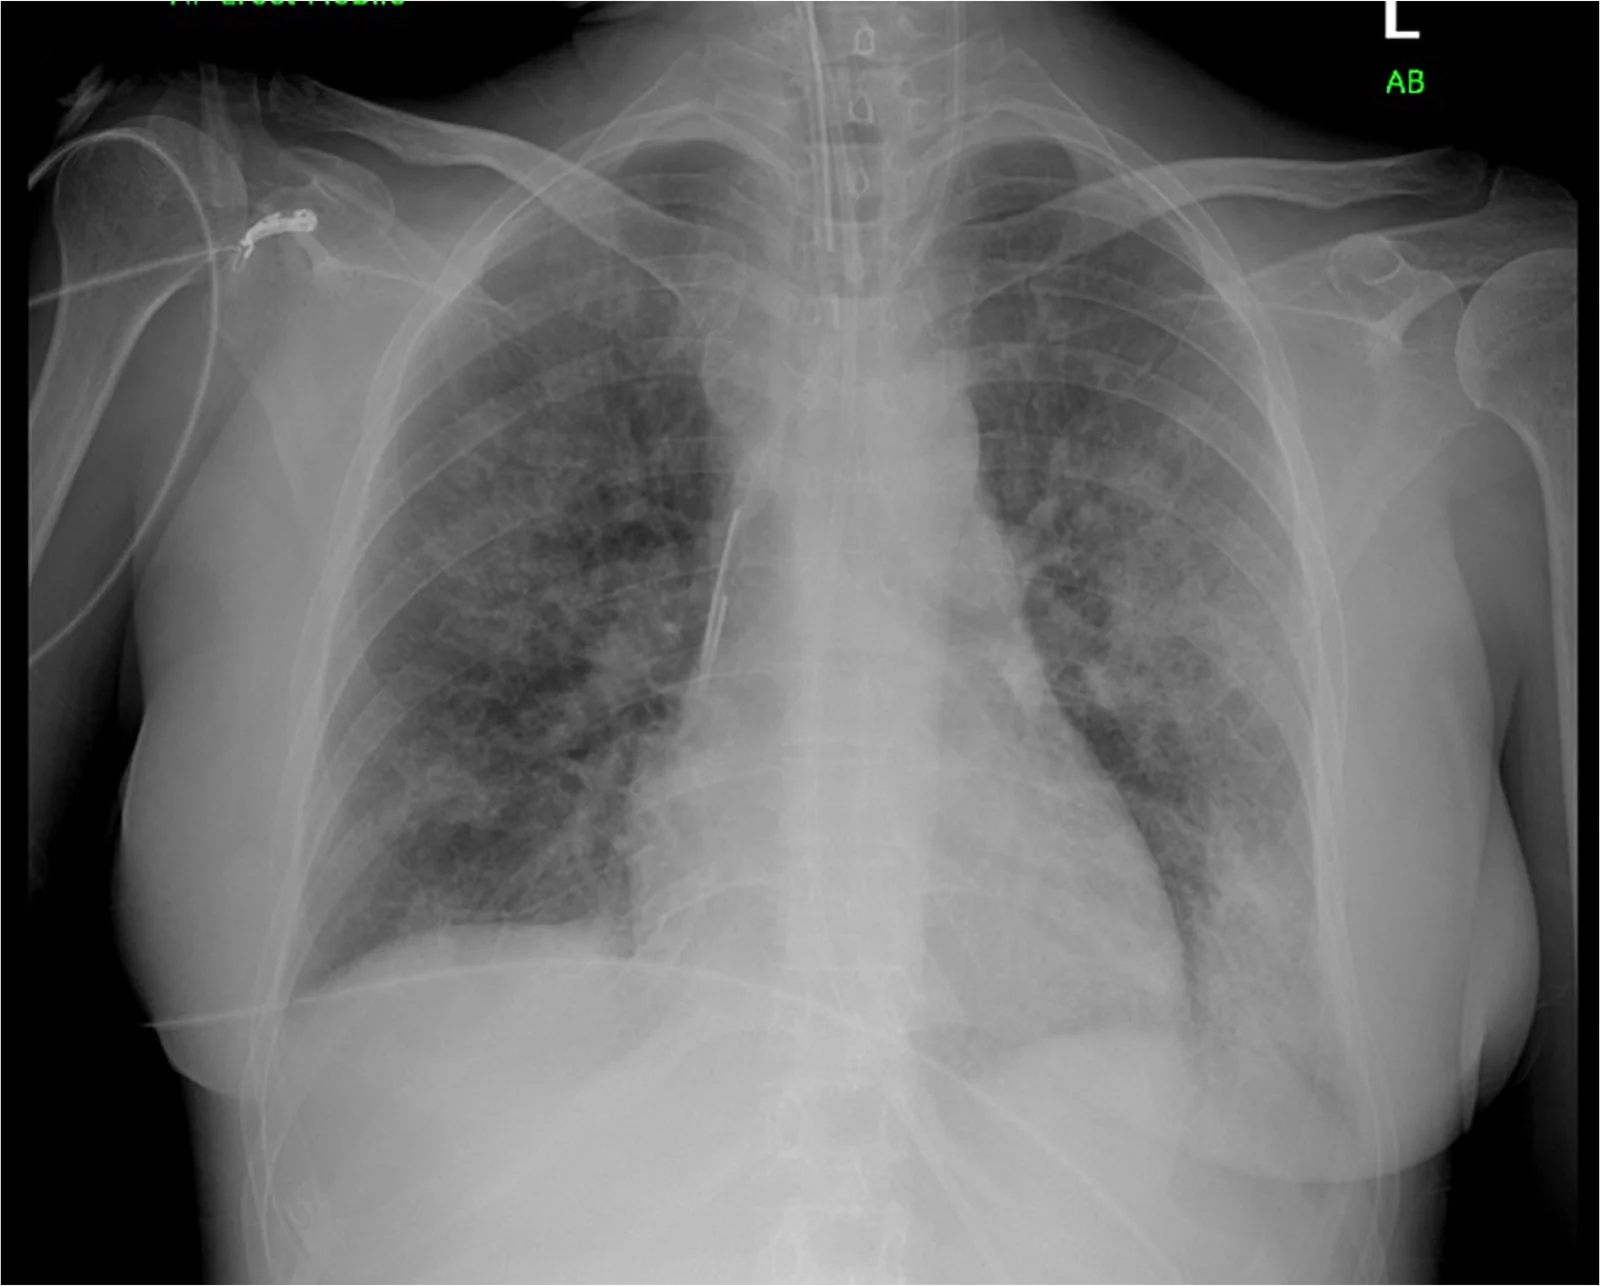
Chest X-ray revealing bilateral infiltrates suggestive of ARDS.
